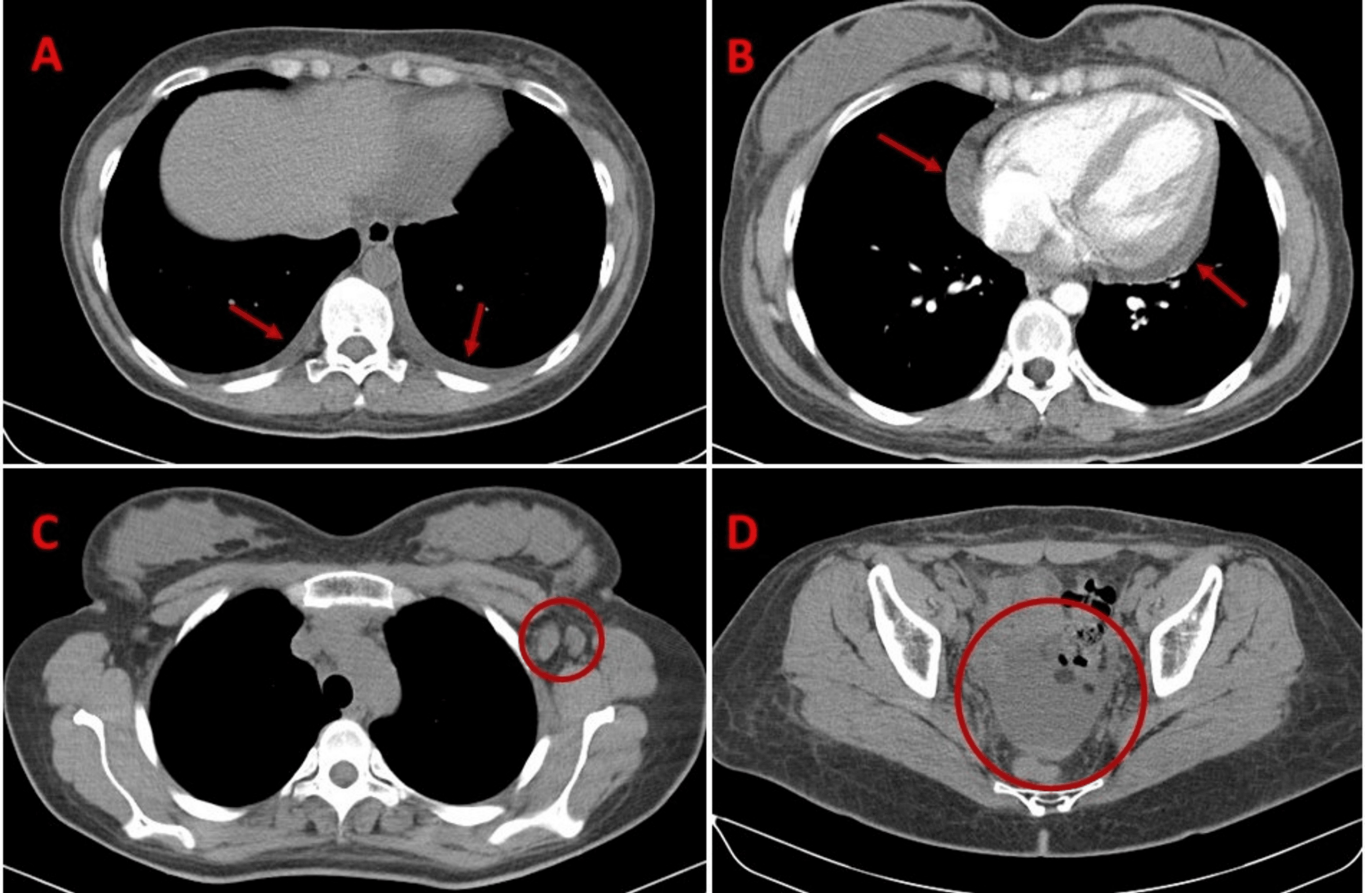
CT-scan findings on admission. These images show the CT-scan findings on admission.  A: shows the pleural effusion (marked by the arrows); B: shows the pericardial effusion (marked by the arrows); C: shows the axillary lymph nodes (highlighted by the circle); D: shows small volume ascites (highlighted by the circle).
